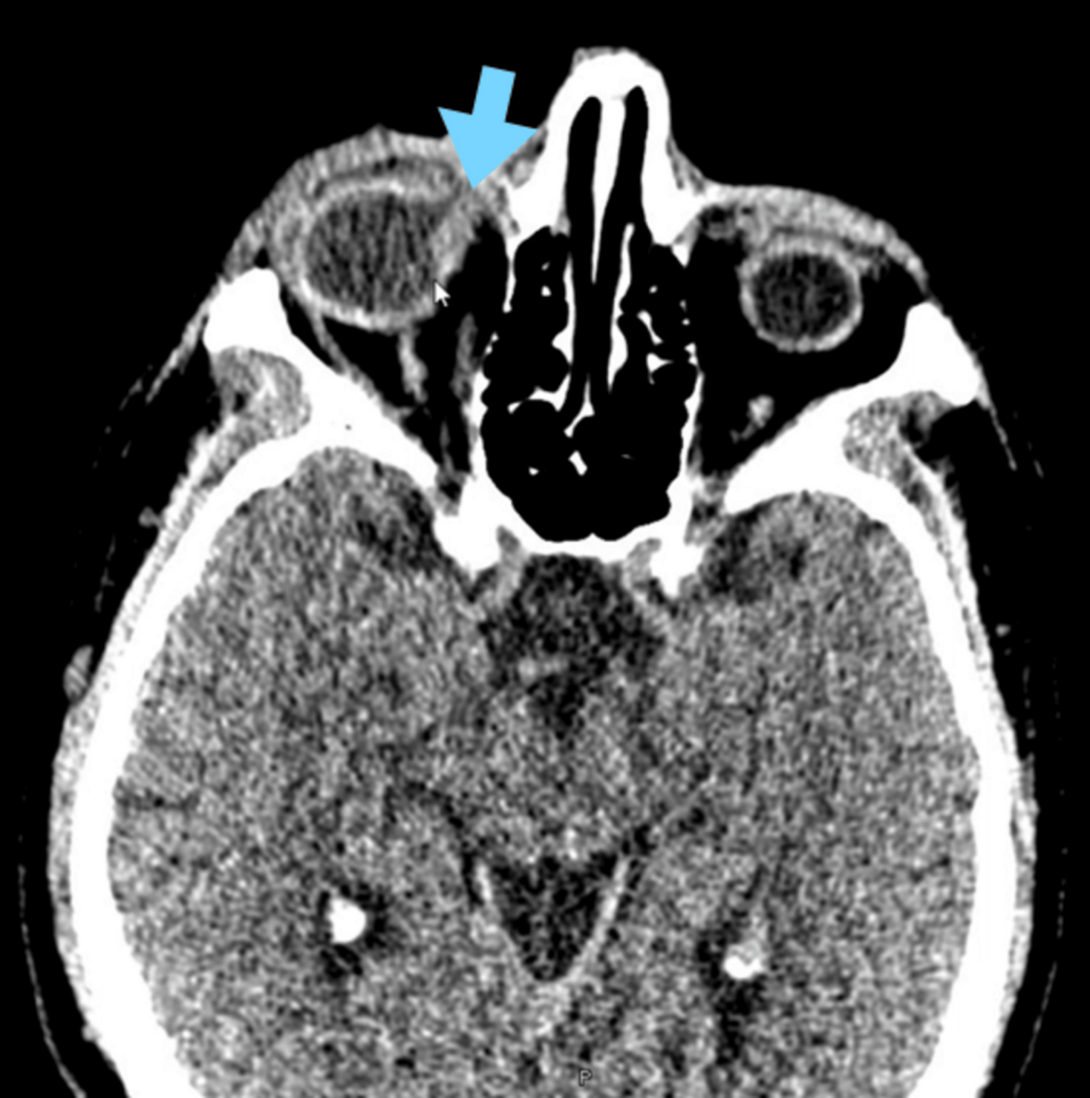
CT scan A vitreous-conjunctival fistula is observed at the arrow. CT: computed tomography
